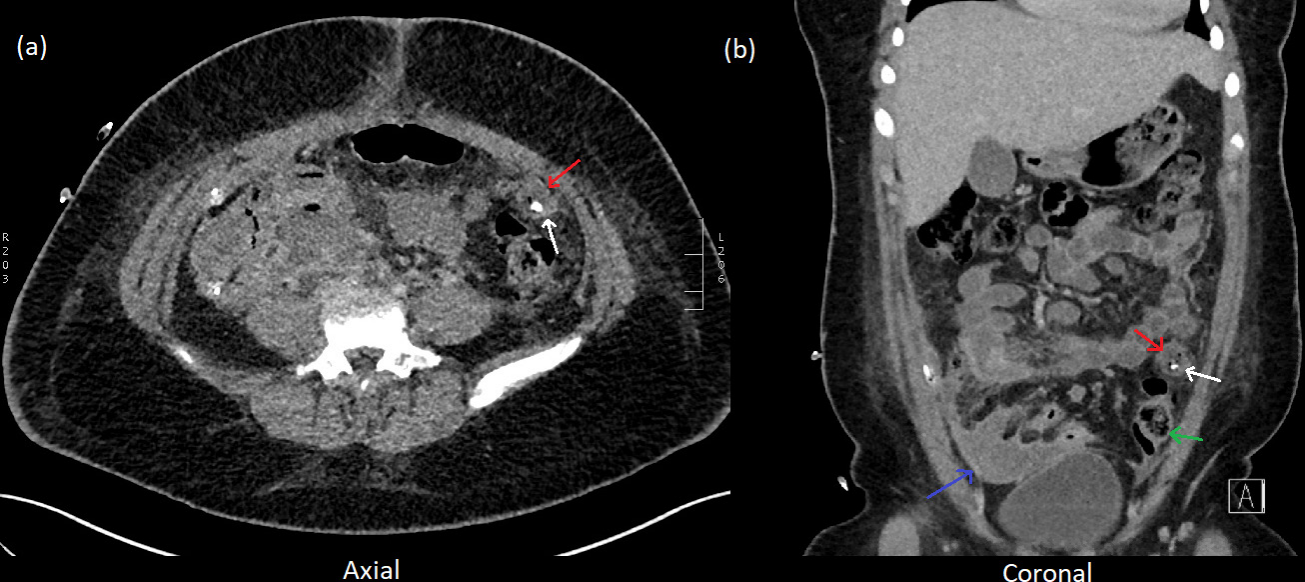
Day eight post op CT ordered for investigation of post-operative sepsis
